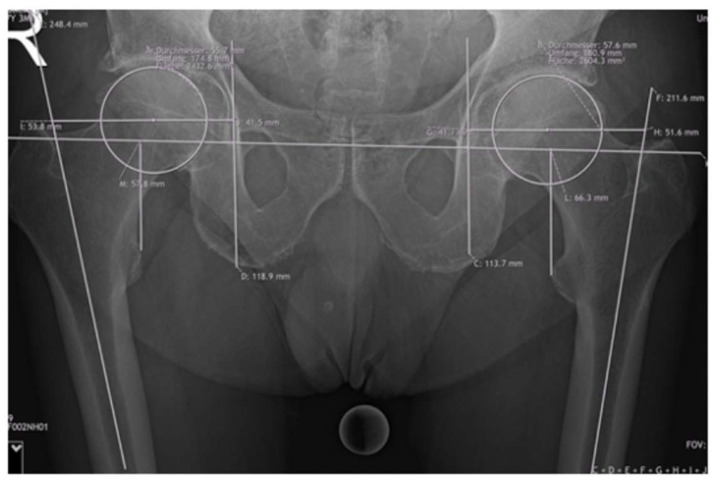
Measurements of FO, AO, HO, and leg length on a preoperative radiograph.
